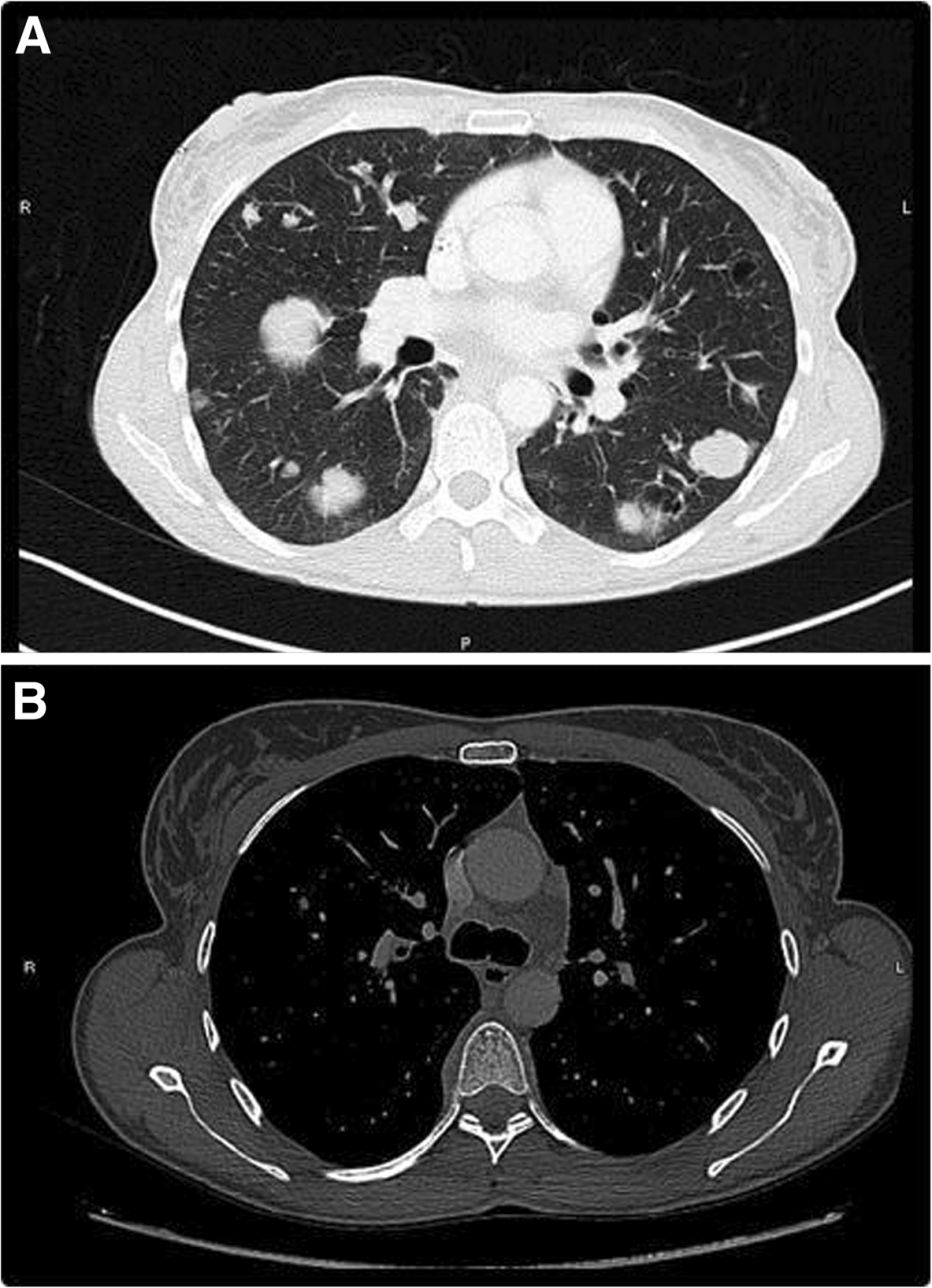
Computed tomography scan at the initial diagnosis. (A) Parenchymal sequence. (B) Mediastinal sequence.
